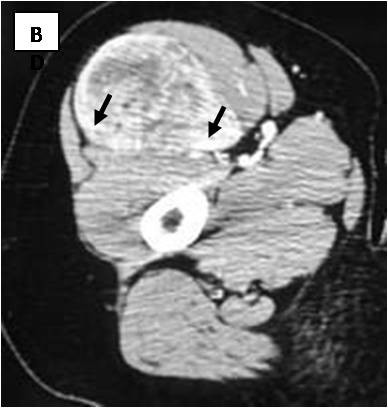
Axial contrast-enhanced CT scan: Delineation of vessels (black arrows and arrowheads) is apparent on enhanced images.
